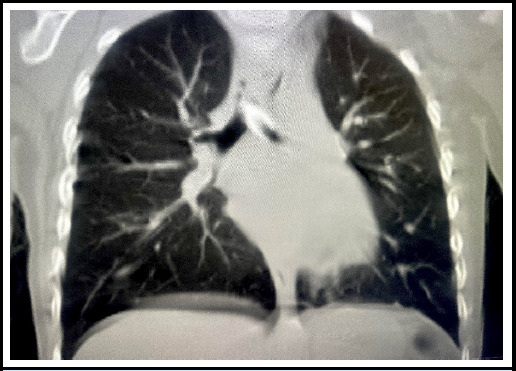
Computed Tomography (CT) scan of Chest revealing foreign body in left main bronchus.
